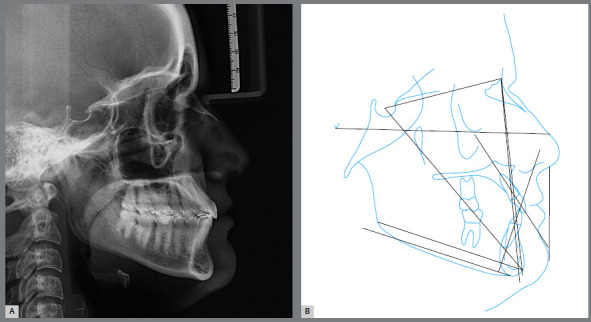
Cephalometric radiograph and tracing10 years after the end of treatment.
